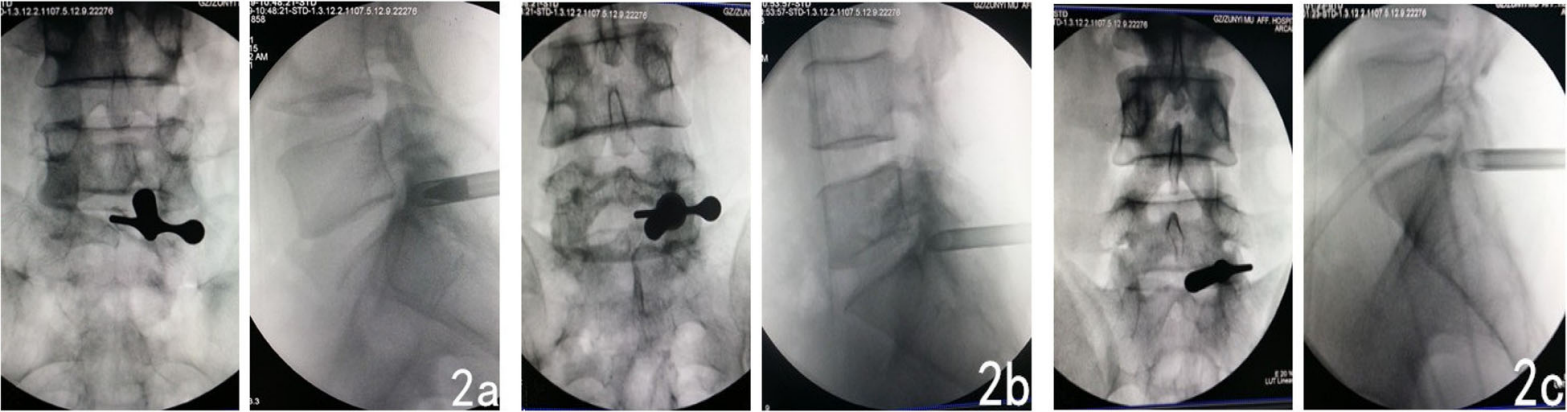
Anteroposterior X-ray image of intraoperative cannulas: a. shoulder type; b. ventral side type; c. axillary type
